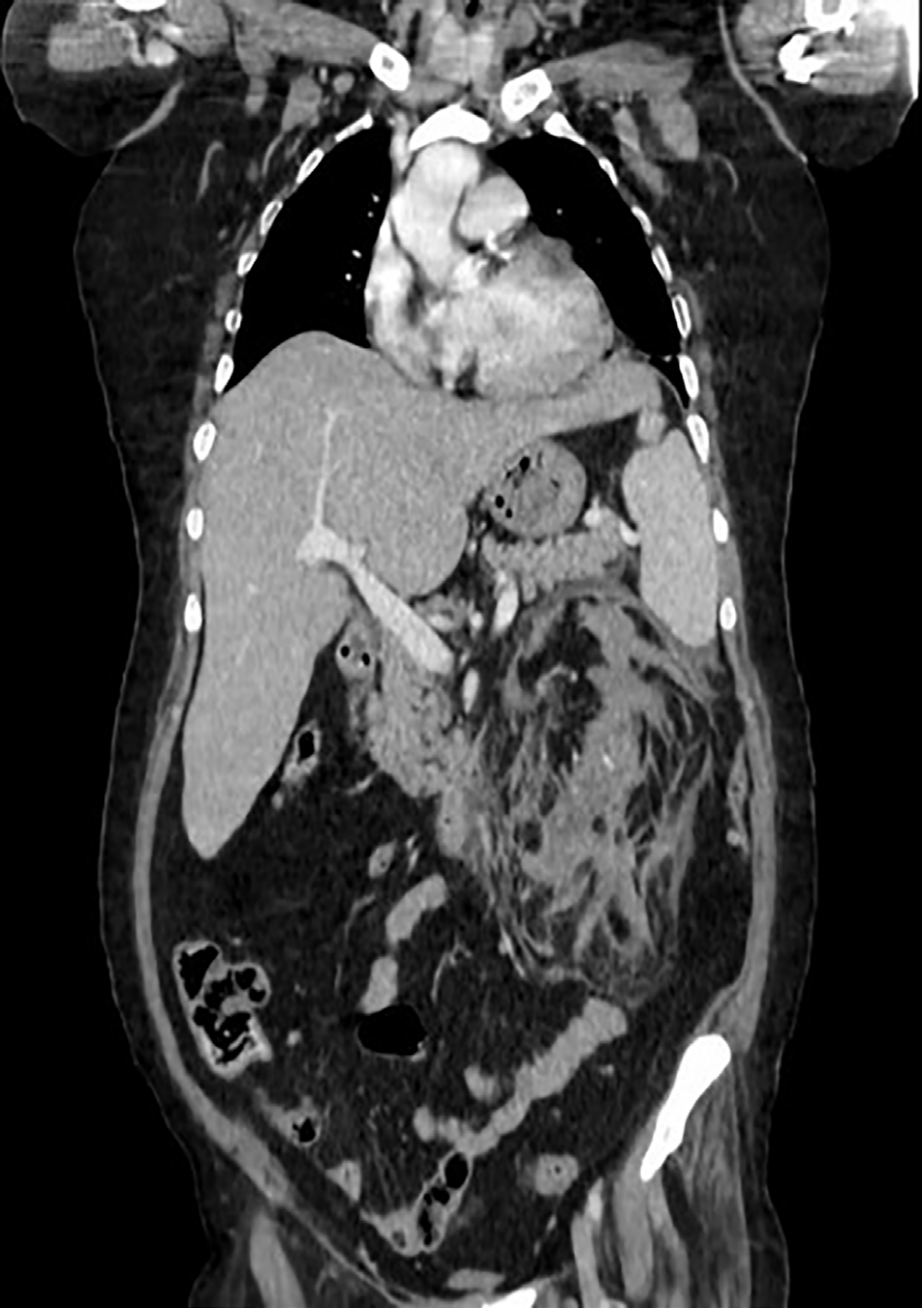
Coronal CT scan section showing the left perirenal intraparenchymal hematomas with a subcapsular collection of up to 1.8-cm thick with hematic collection throughout the renal compartment reaching a diameter of more than 9×4 cm.
